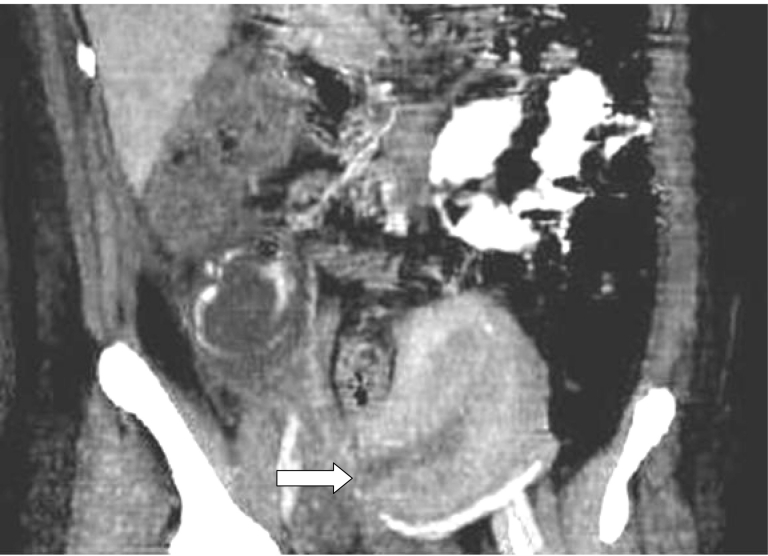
Enhanced reconstructed CT image (oblique coronal) shows the inconsistency of the right lateral wall of the myometrium (arrow), a finding indicative of perforation or rupture. The retroperitoneal lesion, with air bubbles and peripheral enhancement is also visible.
